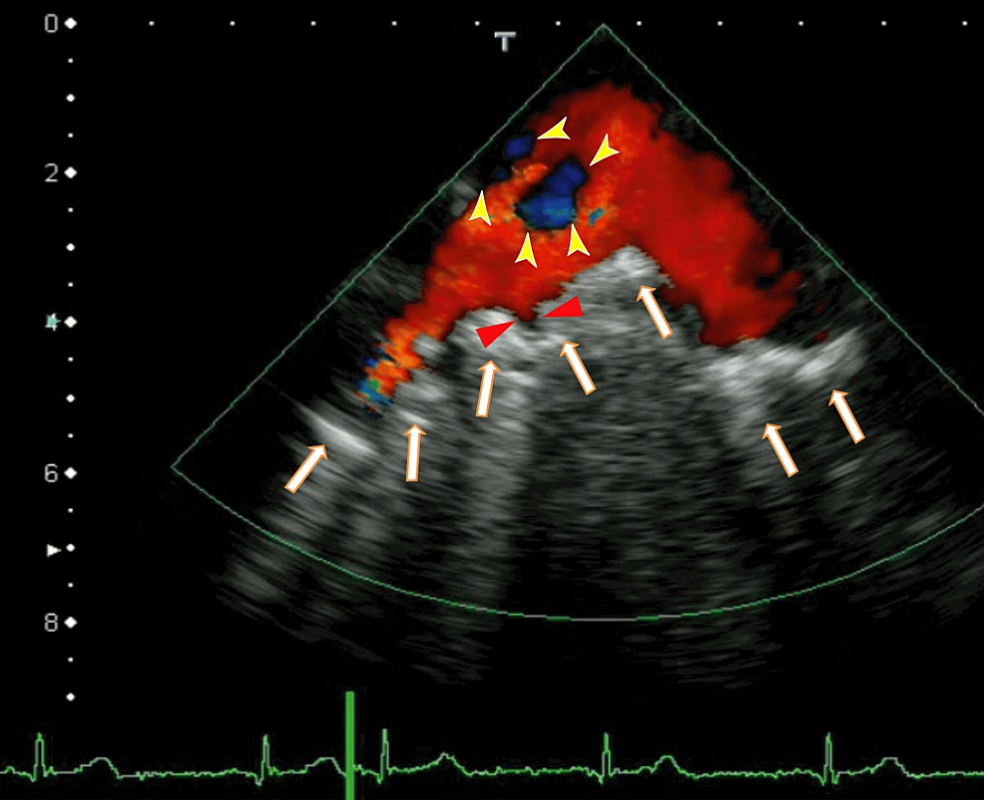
Transesophageal echocardiography (TEE) showing thrombi in the left atrium (LA) The thrombi were located between the ostia of the left upper pulmonary vein (LUPV) and the ostia of the right upper pulmonary vein (RUPV), and the location was the anterior wall of the LA (arrows). Blood influx from the LUPV and RUPV around the thrombus is shown in red. Dark thrombi were identified as a lack of red blood flow from the RUPV (yellow arrowheads). The left atrium diverticulum (LAD) can be identified (red arrowheads). LA: left atrium; LUPV: left upper pulmonary vein; RUPV: right upper pulmonary vein
